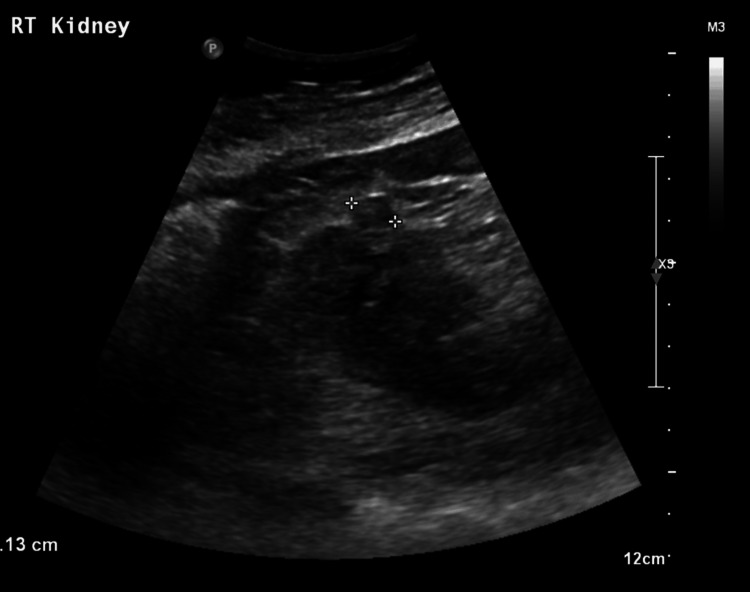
Ultrasound of the right kidney Ultrasound of the right kidney demonstrating the renal lesion.
